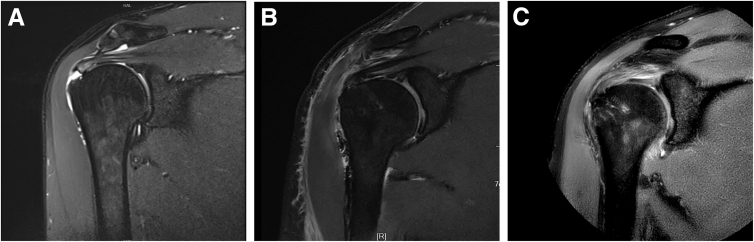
Partial-thickness bursal-sided rotator cuff tear of the right supraspinatus tendon in a 45-year-old male patient. (A) Oblique coronal magnetic resonance imaging (MRI) shows a partial-thickness bursal-sided rotator cuff tear preoperatively. (B) Oblique coronal MRI obtained at 1 day postoperatively. (C) Oblique coronal MRI obtained at 3 months postoperatively.
